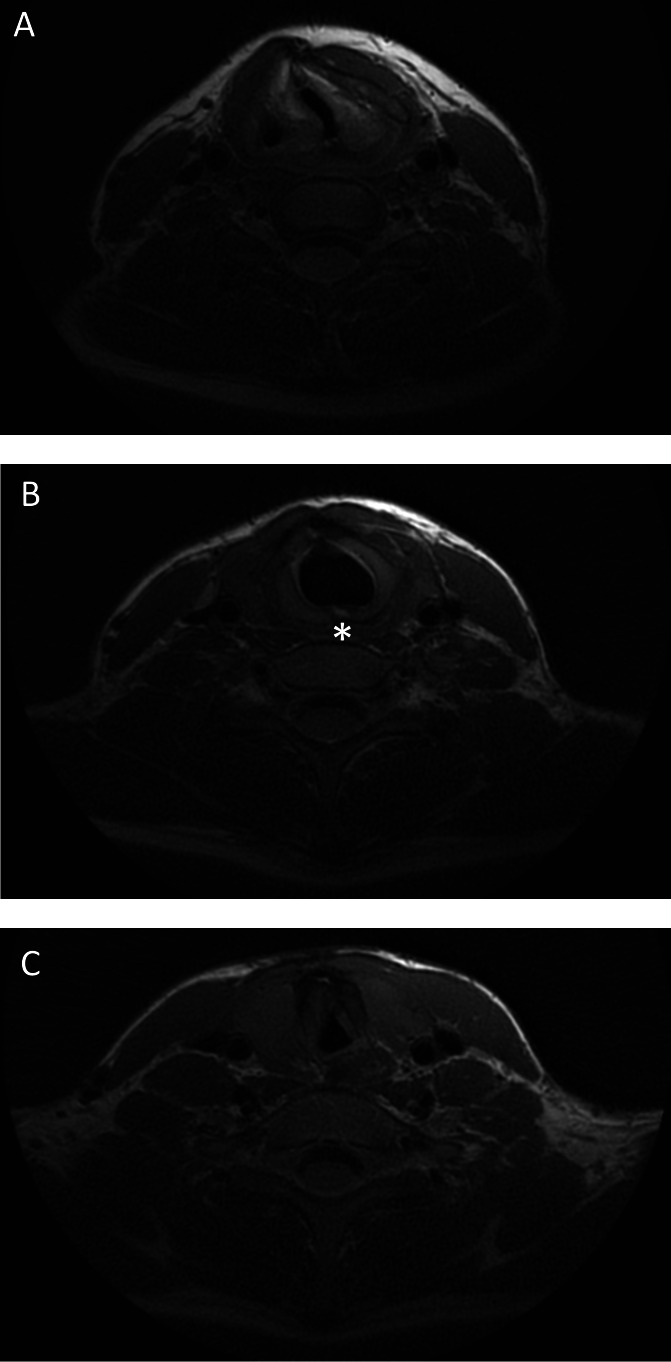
Example of static MRI image of a patient with a history of LTS after prolonged intubation due to prematurity having undergone both an SS-LTR and ds-LTR, showing deviation and thickening of the vocal cords (A), asterisk indicating the posterior cartilage graft placed during LTR (B) and the A-frame deformation of the trachea (C). ds-LTR, double stage laryngotracheal reconstruction; LTS, laryngotracheal stenosis; SS, single stage.
